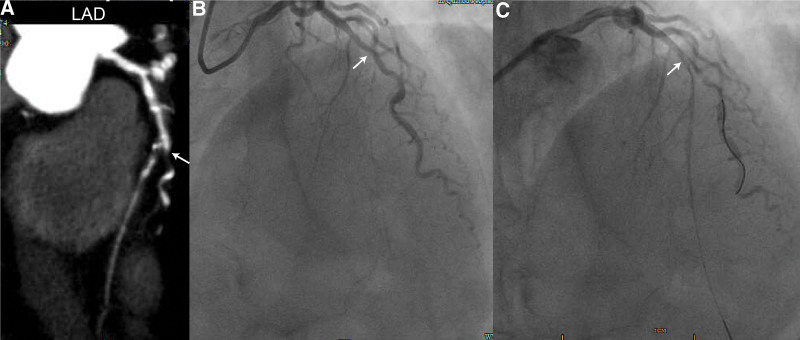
(A) Coronary CT angiography demonstrates mixed and noncalcified plaques in the LAD with mild-to-severe luminal stenoses across segments. (B) Digital subtraction angiography (DSA) shows approximately 60% ostial LAD stenosis and complete occlusion distal to the proximal–mid segment. (C) Post-balloon angiogram reveals residual moderate-to-severe proximal stenosis and severe stenosis near the second diagonal branch origin. CT = computed tomography, DSA = digital subtraction angiography, LAD = left anterior descending.
